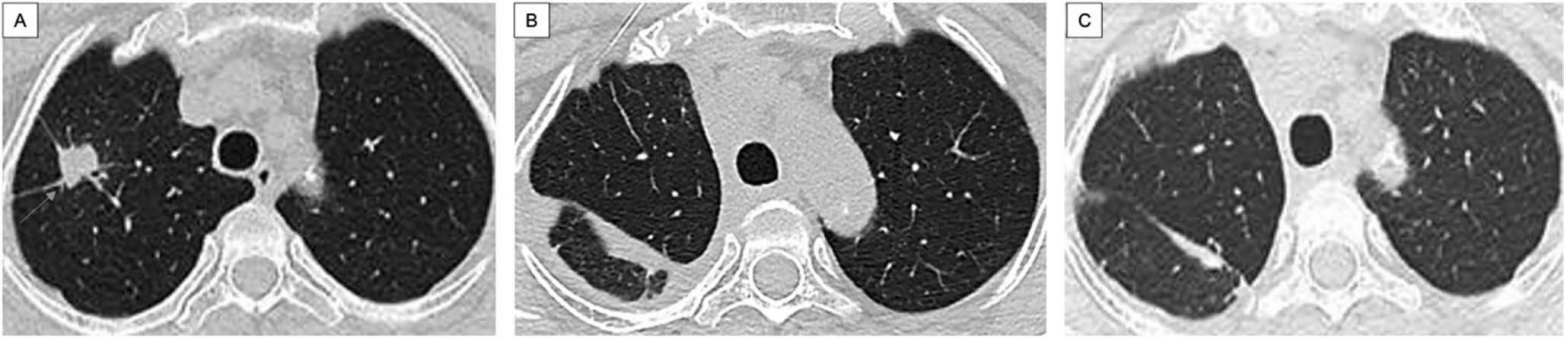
CT scan of the right lung at recurrence and during post‐treatment follow‐up. (A) Enlargement of the right upper lobe tumor, indicating disease progression. (B) One month after right upper lobectomy, no residual or secondary pulmonary lesion was observed; a small right pleural effusion was present. (C) One month after completing four cycles of adjuvant chemotherapy, no new lesions were detected.
